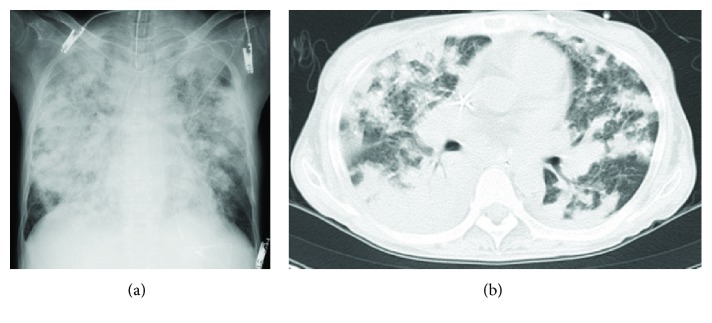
Preoperative chest radiography (a) and computed tomography (b) showing bilateral diffuse consolidation.
